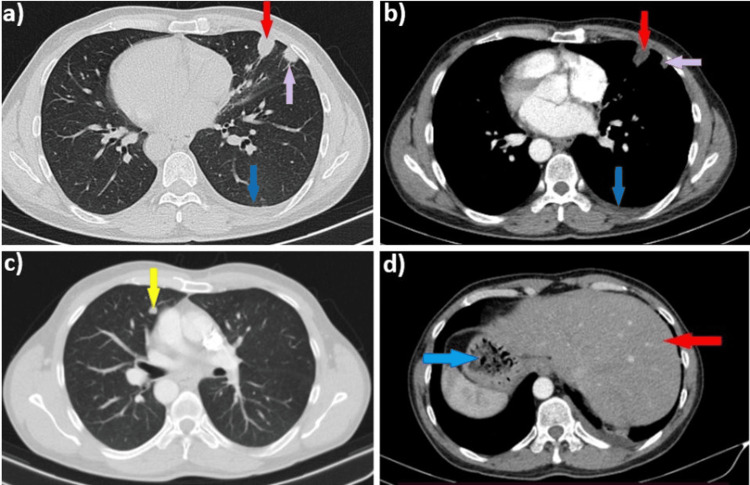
Chest CT scan showing subpleural nodular densifications, pleural effusion, and a left paracardiac micronodule
